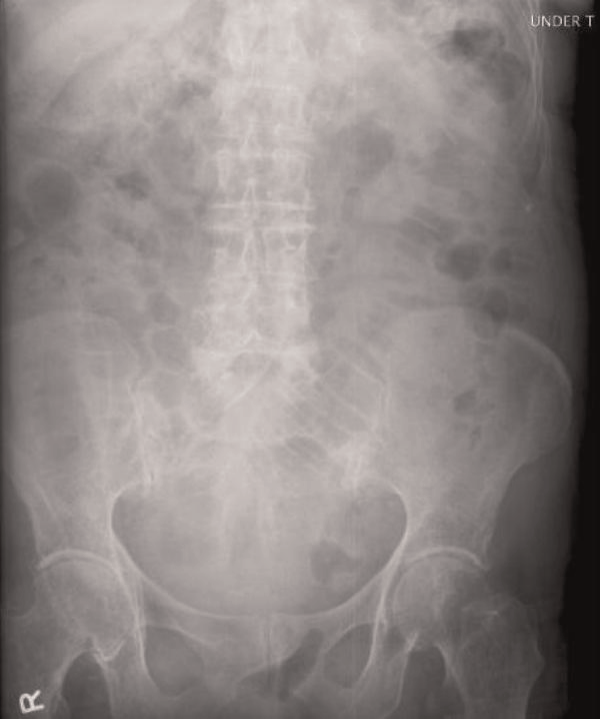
Plain radiograph of the abdomen.
